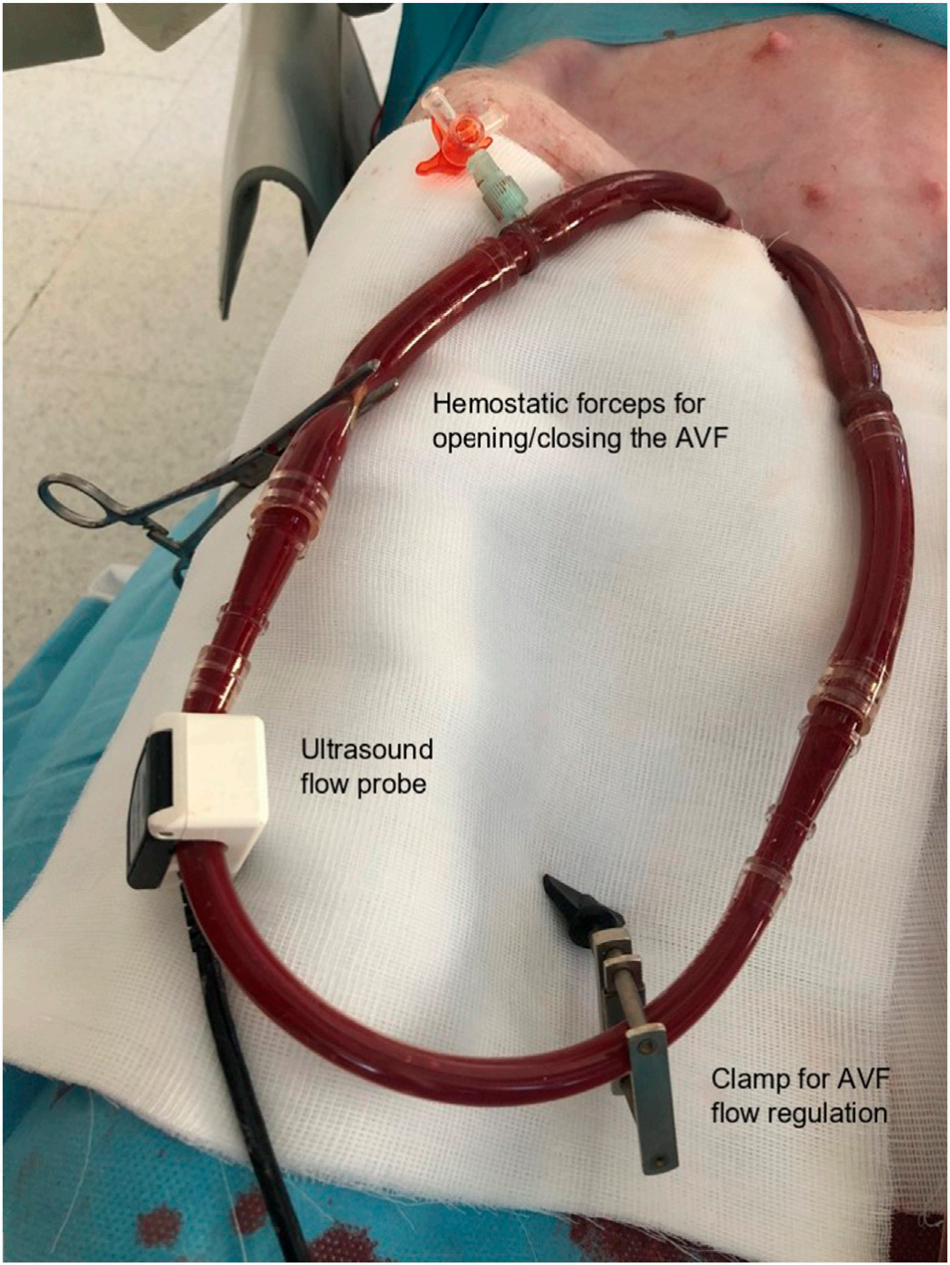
Aortocaval arteriovenous fistula created by the connection of two high-diameter ECMO cannulas. Special clamp to regulate the AVF blood flow volume was placed before connecting the ECMO cannulas (in lower right corner). Continuous measurement of AVF flow was performed using an ultrasound probe (arrow) (Transonic, United States). This photo documents clamping of the fistula circuit by the hemostatic forceps for the observation of the hemodynamic stabilization in the final phase of the experiment.
